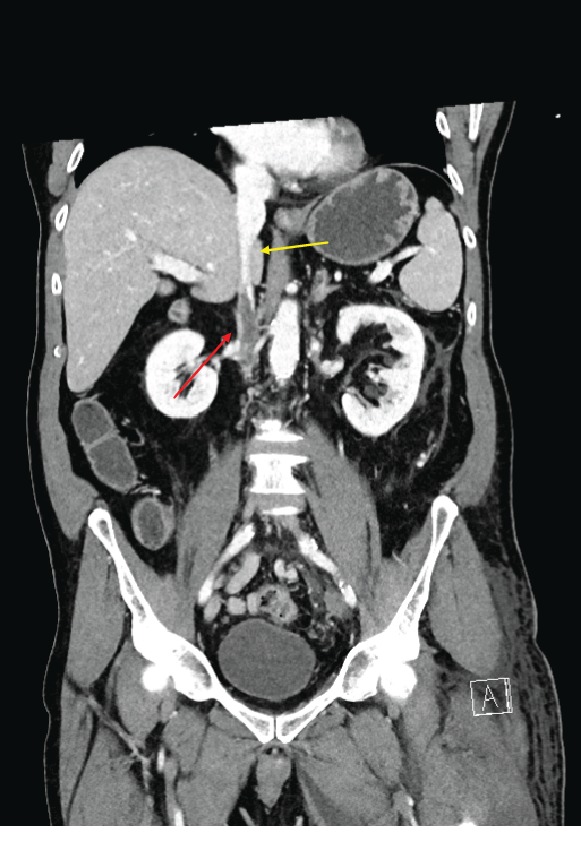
Angio-CT showing inferior vein cava thrombosis (red arrow) and inferior vein cava hypoplasia (yellow arrow).
